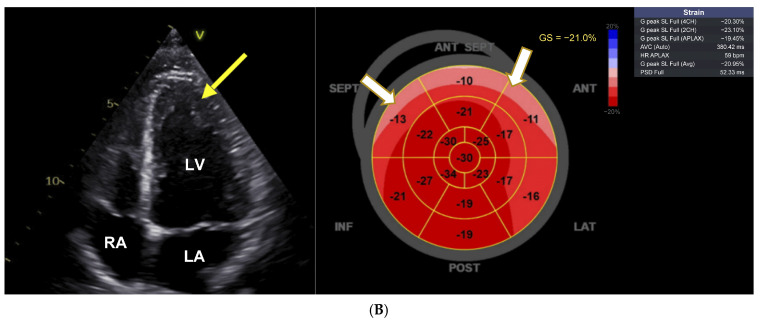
Pronounced cardiac anomaly showing features of hypertrophic cardiomyopathy. (A) Echocardiographic changes with apical hypertrophy and apical sparing pattern with decrease in strain in basal segments (white arrows). LA = left atrium, LV = left ventricle, RA = right atrium; white arrow points to left ventricular hypertrophy. Average GLS as shown on the right side is diminished and shows apical sparing (white arrows). (B) Reversal of left ventricular hypertrophy and apical sparing pattern after dose reduction of venlafaxine. LA = left atrium, LV = left ventricle, RA = right atrium; yellow arrow points to left ventricular hypertrophy. Average GLS as shown on the right side has now almost completely normalized (−21.9%), with some remaining decrease in basal septal, anteroseptal, and anterior segments (white arrows).
